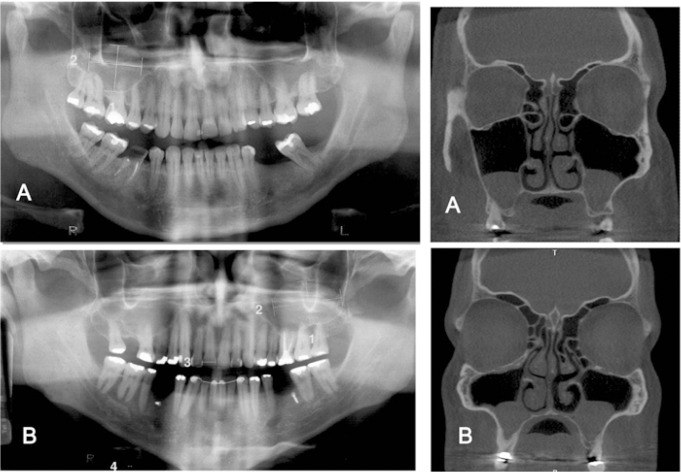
A) Control panoramic radiograph with MRCMS in right side and coronal CBCT reconstruction confirming MRCMS in right side and showing another MRCMS in left side. B) Control panoramic radiograph with MRCMS in left side and coronal CBCT reconstruction of same patient with bilateral MRCMS.
